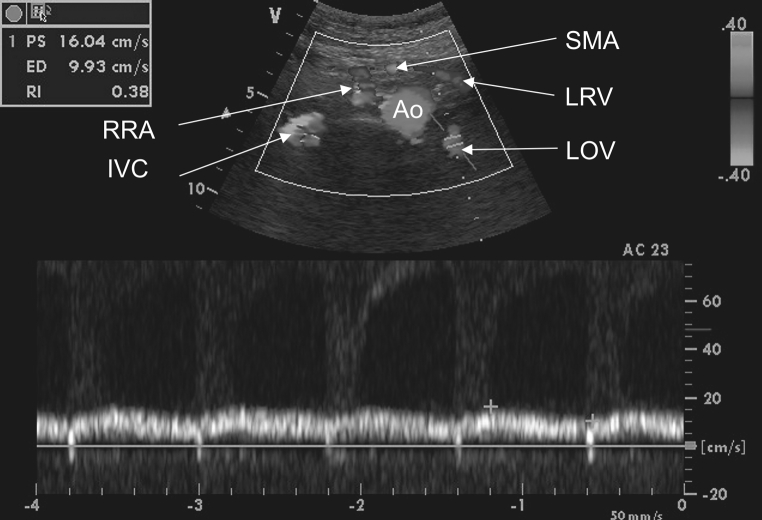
Color Doppler ultrasonography abdominal vessels. *Ao* abdominal aorta, *IVC* inferior vena cava, *LOV* left ovarian vein, *LRV* left renal vein, *SMA* superior mesenteric artery, *RRA* right renal artery
